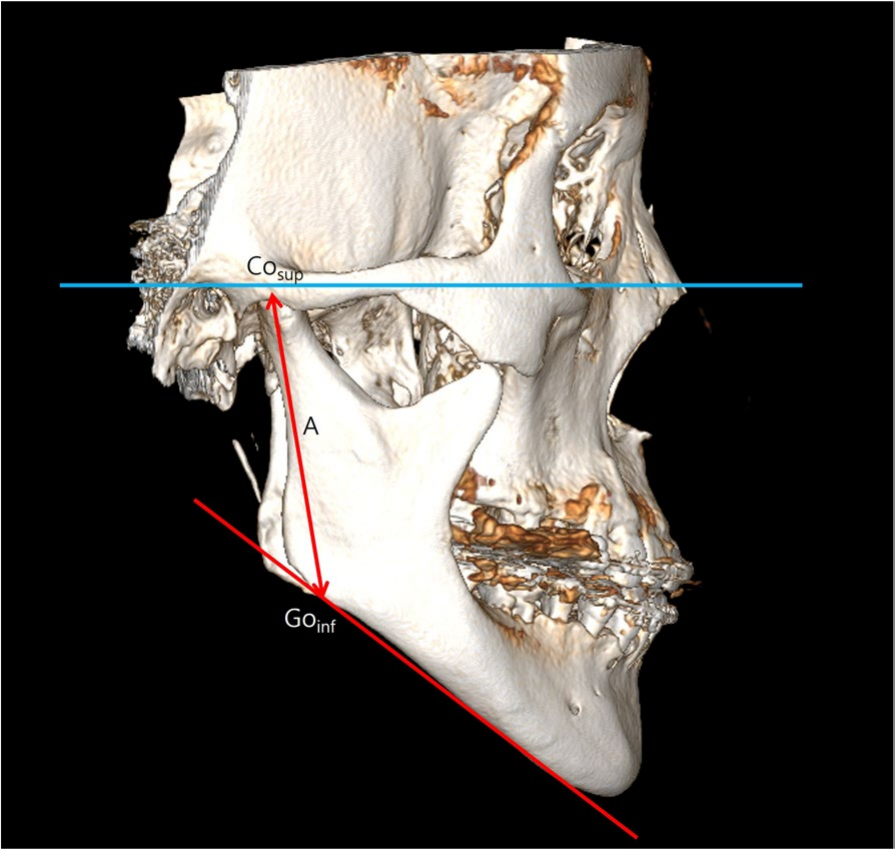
Definition of mandible ramus height through 3D-CBCT. After aligning with the FH plane, the distance is the straight line between the Cosup (the most superior point of the condyle head) and the Goinf (the tangent of the inferior border of the mandible meets the mandibular angle)
